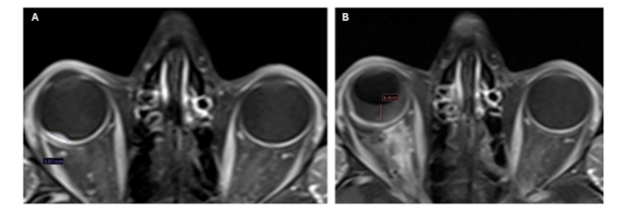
(A) MRI orbit (Day 0) showed enhancing plaque along the posterior-lateral wall of his right globe. (B) MRI orbit (Day 1,123) showed new right optic nerve enhancement.
